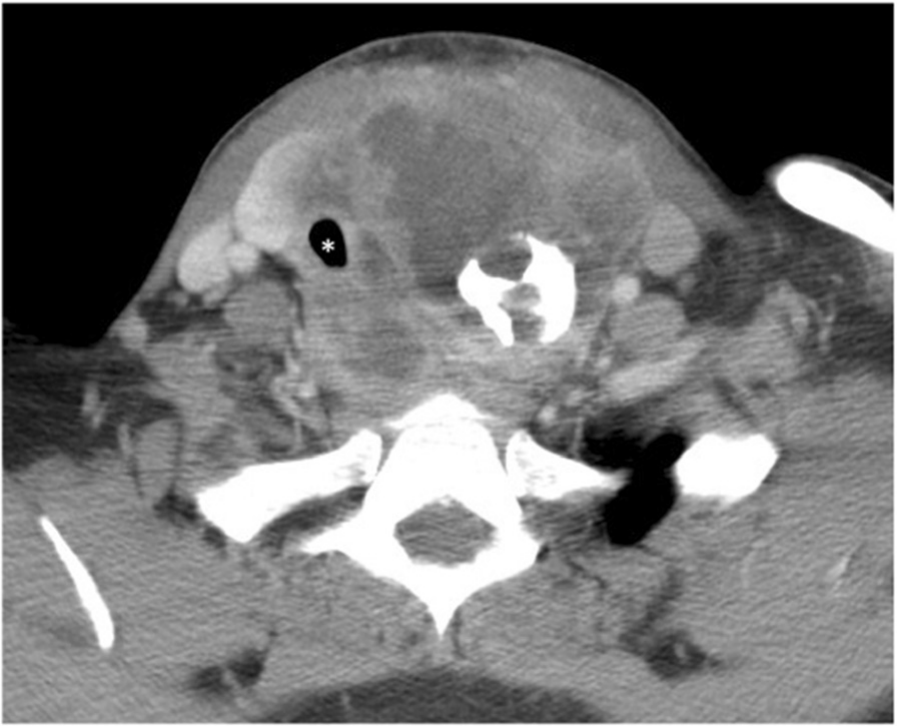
Computer tomography (CT) of the neck revealing a 9 × 12 cm extensively infiltrative mass. * = trachea.
